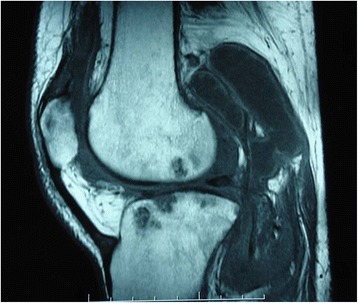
Magnetic resonance imaging showed signs of osteomyelitis in the right femur and tibia
